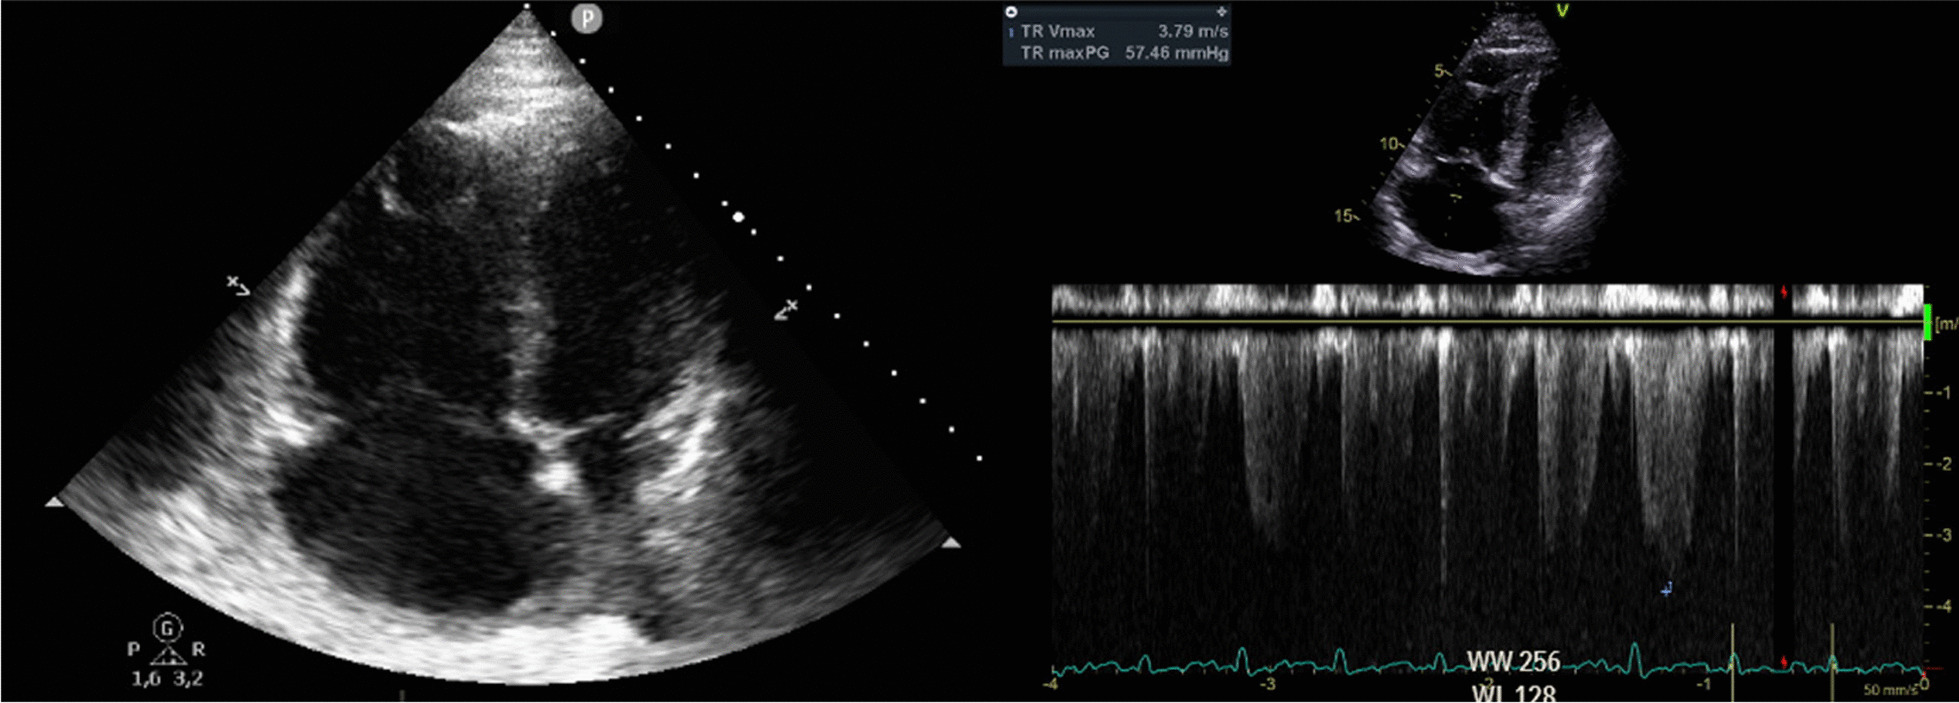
Echocardiography showing acute right heart dilatation
